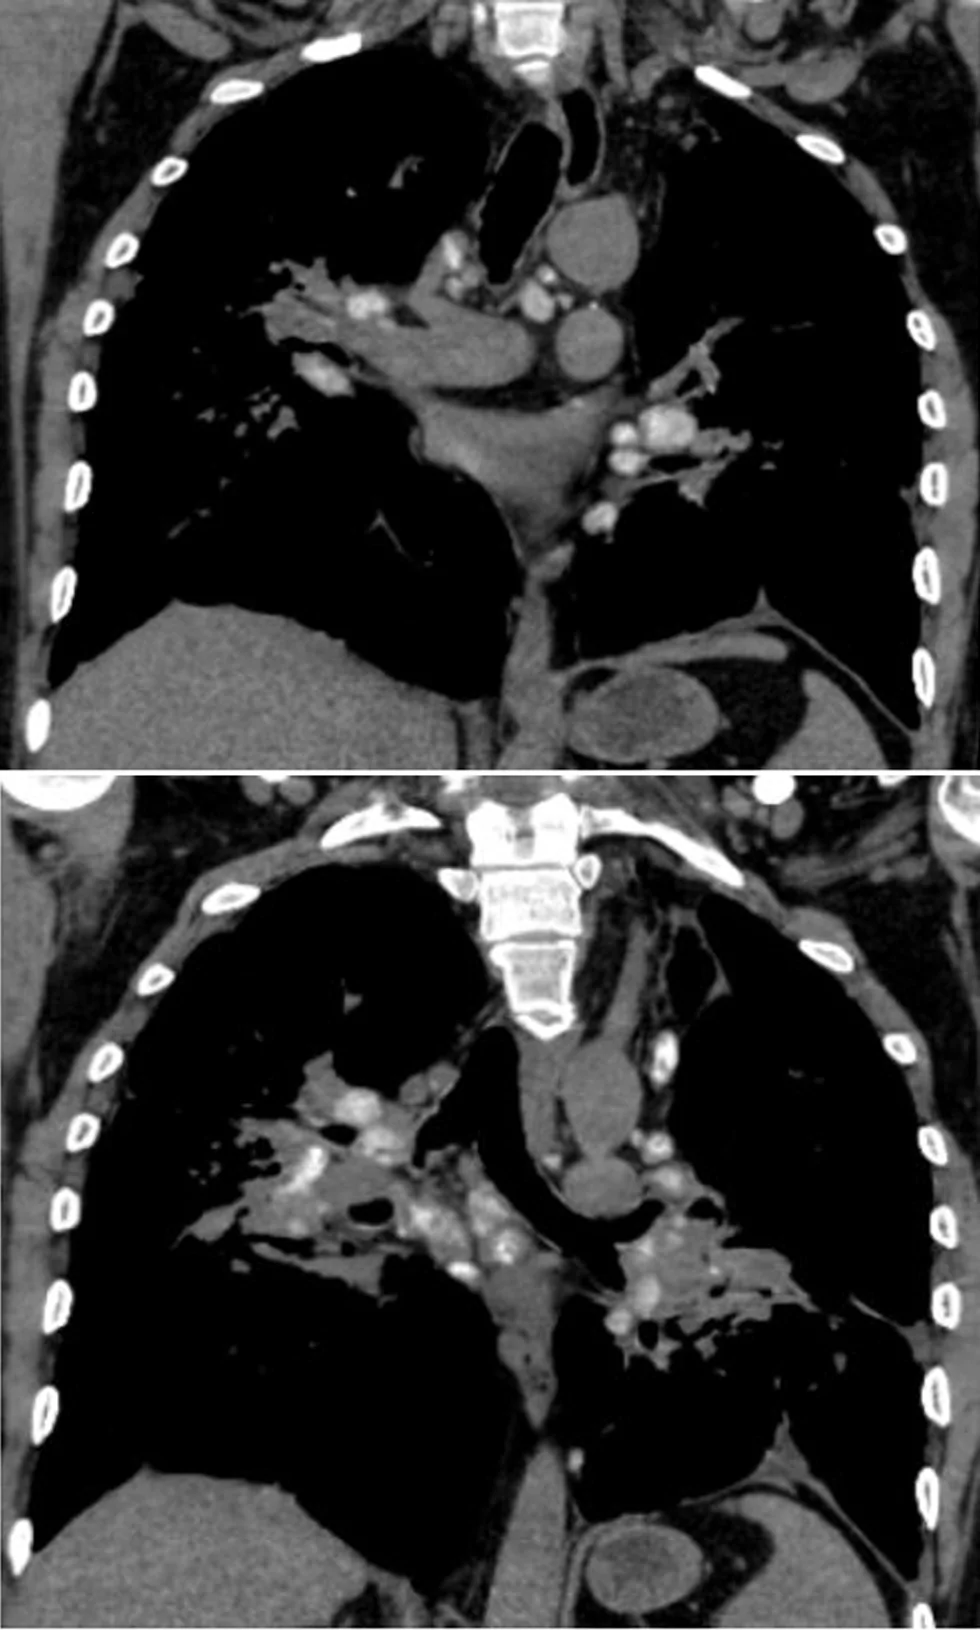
Coronal HRCT image (mediastinal window) demonstrating multiple enlarged mediastinal and hilar lymph nodes involving the upper and lower paratracheal, subaortic, pulmonary, subcarinal, and paraaortic stations. HRCT: high-resolution computed tomography.
